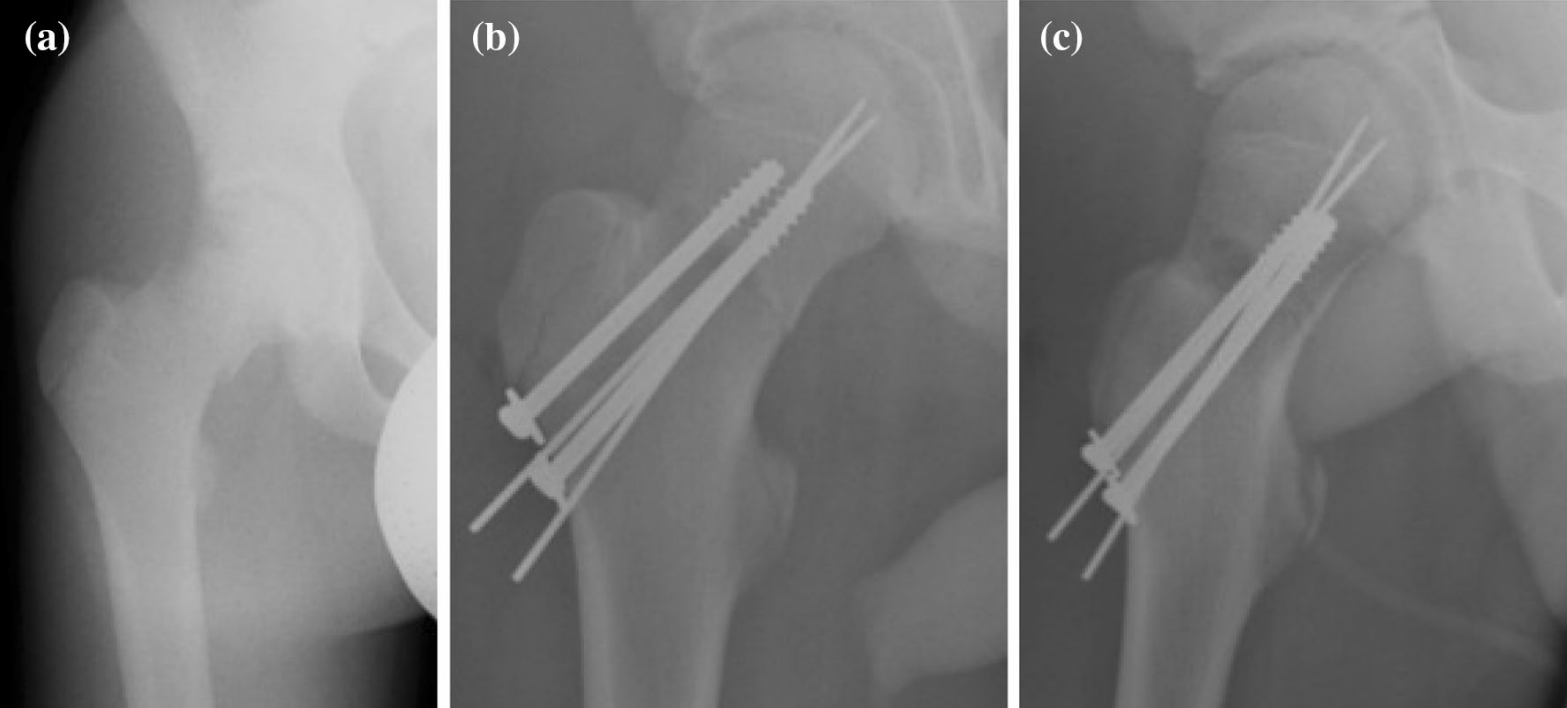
Child’s intracapsular femoral fracture a anteroposterior X-ray, b Osteosynthesis with two lag spongious screws and two Kirschner wires through the epiphyseal zone (anteroposterior X-ray), c Osteosynthesis with two lag spongious screws and two Kirschner wires through the epiphyseal zone (lateral X-ray)
